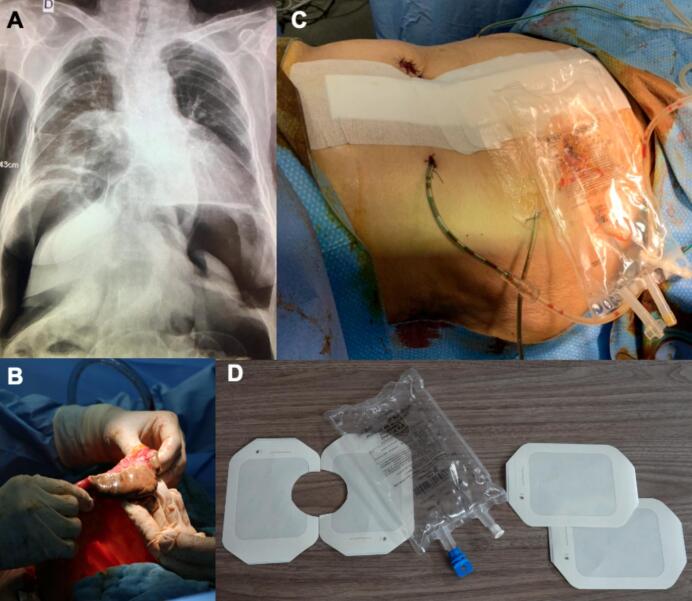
A: Preoperative X-ray showing a large pneumoperitoneum. B: Intraoperative view of the colonic necrosis. C: Immediate postoperative aspect of the stoma. D: Material needed for the stoma supplies – 1 empty 500 cc intravenous perfusion bag and 4 transparent film dressings.
